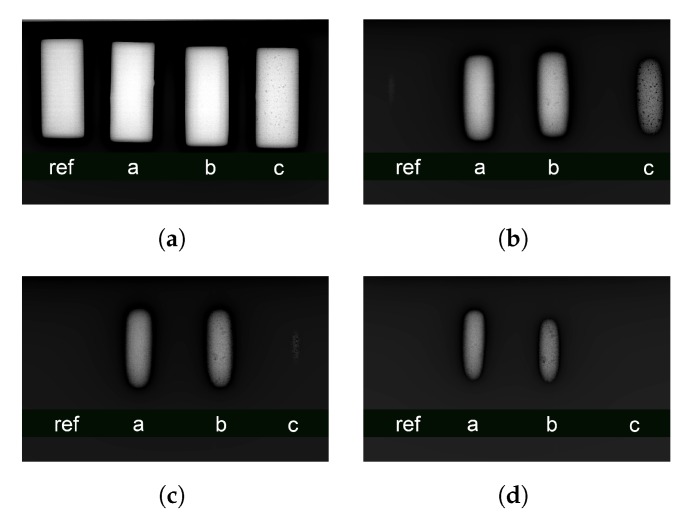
Radiographies on the three ternary mixtures (samples a, b, c in Table 2) developed in laboratory compared with the same steel sample for reference. In this case the photon flux was raised by increasing the current intensity to explore the behavior of the screens in a more severe experimental condition. (a) 120 kV; (b) 180 kV; (c) 200 kV; (d) 220 kV.
